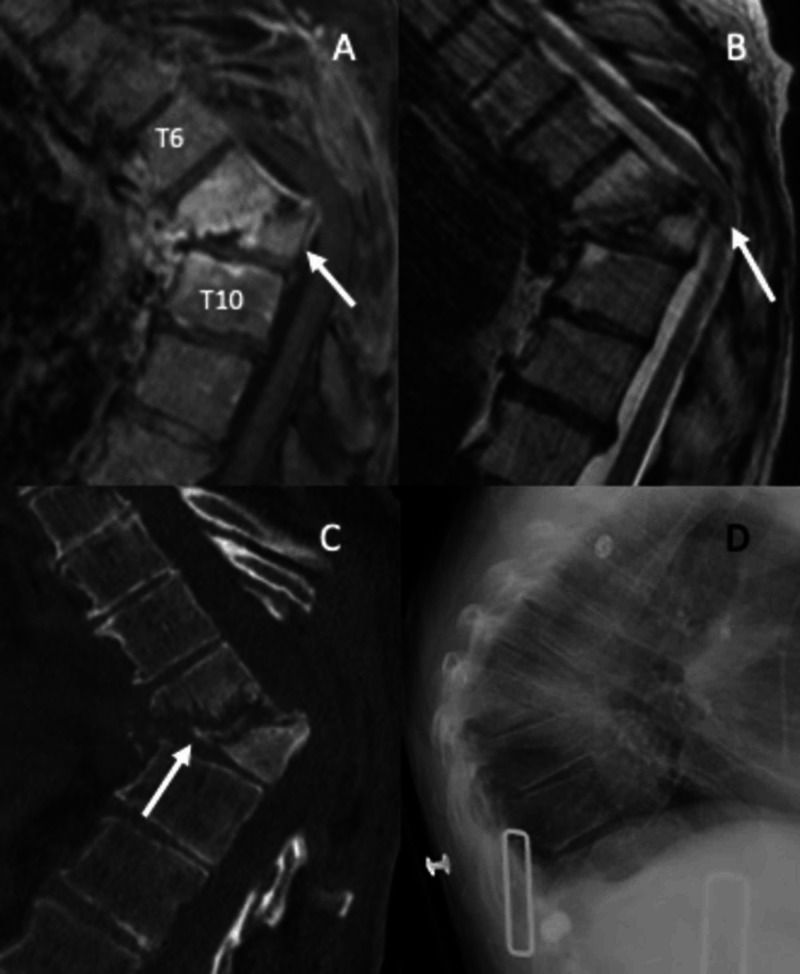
Pre-operative imaging A patient presenting several months after the initial epidural abscess evacuation with progressively worsening back pain and myelopathy. A: sagittal T2 MRI. B: sagittal T1 with contrast MRI. C: sagittal CT scan. D: sagittal XR upright in brace. Progression of osteomyelitis/discitis is evident with severe kyphotic deformity and destruction of the T7, T8, and T9 vertebral bodies. Arrows: A/B - severe kyphotic deformity showing the severity of spinal cord compression; C - erosive changes within multiple vertebrae consistent with osteomyelitis.
